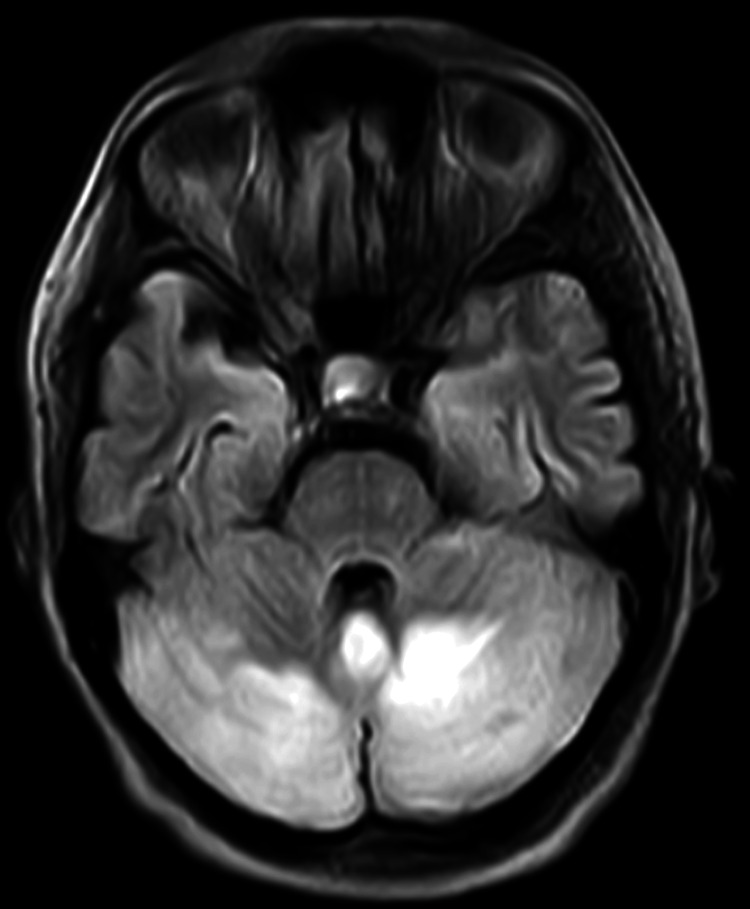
Diffusion restriction involving the cerebellum on MRI fluid-attenuated inversion recovery.
